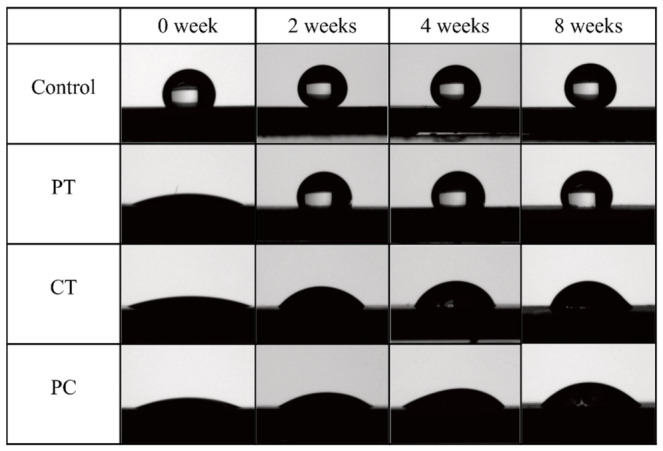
Representative contact angle images of water droplets on gutta-percha surfaces after different surface treatments. PT: atmospheric air plasma treatment; CT: immersion in 100 mM CPC solution; PC: atmospheric air plasma treatment followed by immersion in 100 mM CPC solution.
